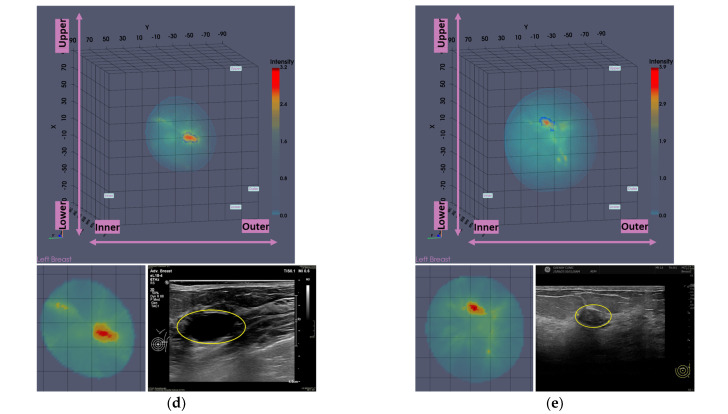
Reference and Wavelia MWBI images for the 4 typical cases of good-quality Wavelia MWBI images with clear characterization as malignant or benign lesions in the Wavelia feature analysis space: (a) Endoscopic camera views of the 4 breasts after positioning in the MWBI scanner—depicted camera views are aligned with the orientation of the MWBI 3D image’s Default View. (b) P003-L IDC—Luminal A. (c) P031-L IDC—Luminal B. (d) P001-L—Cyst. (e) P023-L—Fibroadenoma. (b–e): Wavelia MWBI 3D image’s Default View, with the extracted ROI delineated and superimposed in blue, coronal XY slice of the MWBI image cut at the centroid of the clinically relevant ROI in the MWBI image, and snapshot of the ultrasound scan of the breast provided as reference data for the same lesion in this study.
